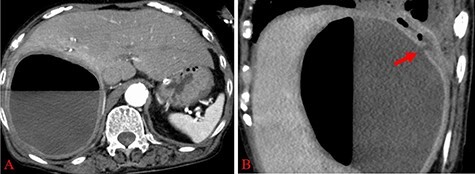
(A) Abdominal enhanced CT reveals a rapid increase of air within the hepatic cyst; (B) Abdominal enhanced CT showing disruption of the continuity of the wall of the hepatic cyst; the fluid component is continuing to the pleural cavity (red arrow).
